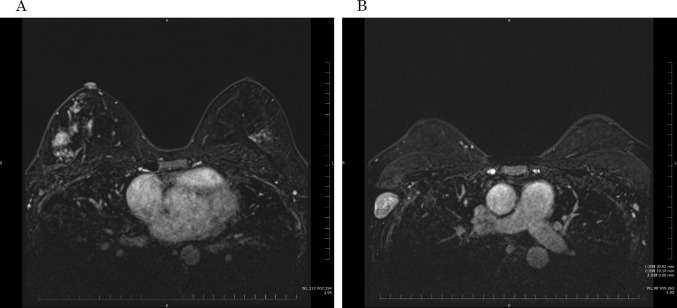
Breast MRI. Right breast nodules (A) and enlarged axillary lymph nodes (B) were noted
